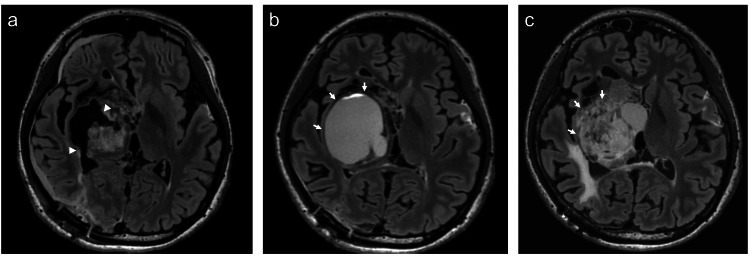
Radiological course following fenestration and radiotherapy (a) Postoperative FLAIR obtained after multiple cyst fenestrations (arrowheads) were performed via a small craniotomy. (b) Follow-up FLAIR one month after surgery demonstrates progressive enlargement of the cystic component despite fenestration (arrows). (c) FLAIR obtained two years after radiotherapy showed stabilization of the cystic component without further enlargement (arrows).
